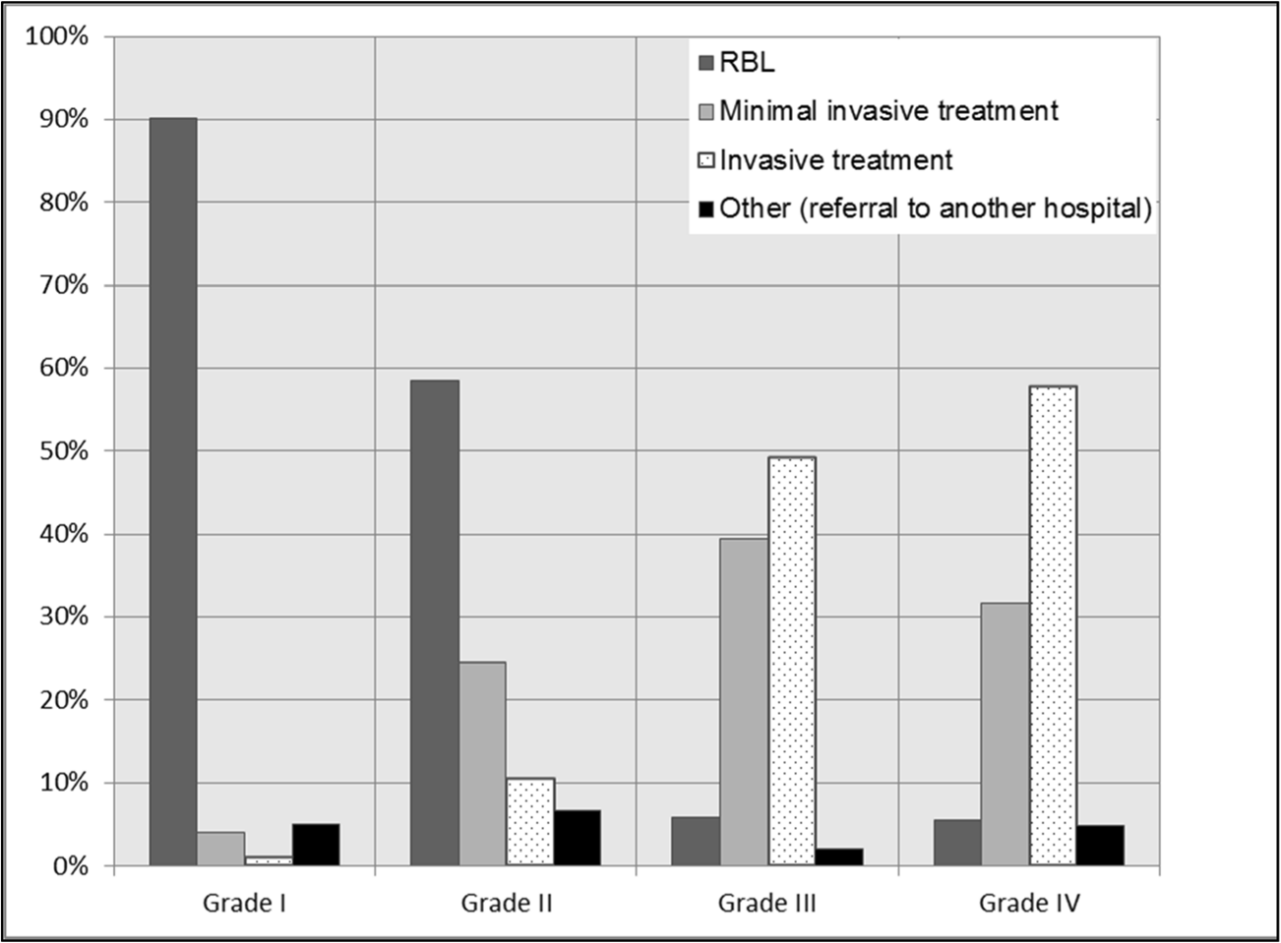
The use of rubber band ligation (RBL), minimal invasive treatment (laser therapy or sutured hemorrhoidopexy or Doppler-guided hemorrhoidal artery ligation (DG-HAL)), or invasive treatment (stapled hemorrhoidopexy or traditional hemorrhoidectomy) for grades I–IV hemorrhoidal disease according to the Goligher classification
